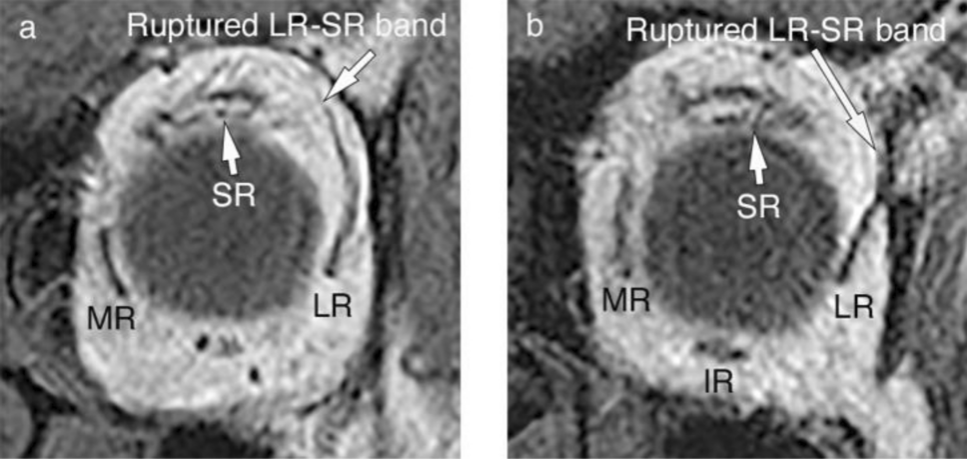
T1-weighted quasi-coronal images of patients with sagging eye syndrome. a Moderate rupture of the lateral rectus (LR)- superior rectus (SR) band with marked LR and medial rectus (MR) muscle sags in the left orbit of patient number 10. b Severe rupture of the LR-SR band in the left orbit of patient number 2. *IR* inferior rectus
